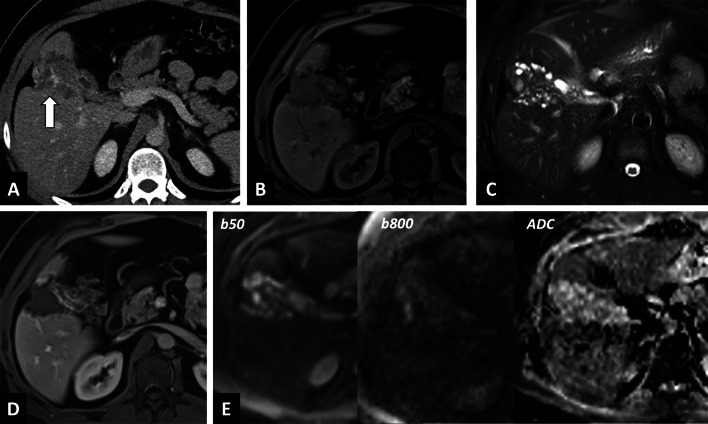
Alveolar echinococcosis in a 57 year old man with an initial suspicion of gallbladder carcinoma. A CT images in the axial plane obtained during the portal phase of enhancement: infiltrative hypodense liver lesion with calcifications infiltrating the gallbladder (arrow). B Fat-suppressed T1-weighted sequence in the axial plane showing hypointensity of the liver lesion. C T2-weighted fat-suppressed MR image in the axial plane showing typical microcysts within hypointense T2 lesions (arrowheads). D Diffusion-weighted imaging showing suppressed hyperintensity on b800 corresponding to high ADC values
